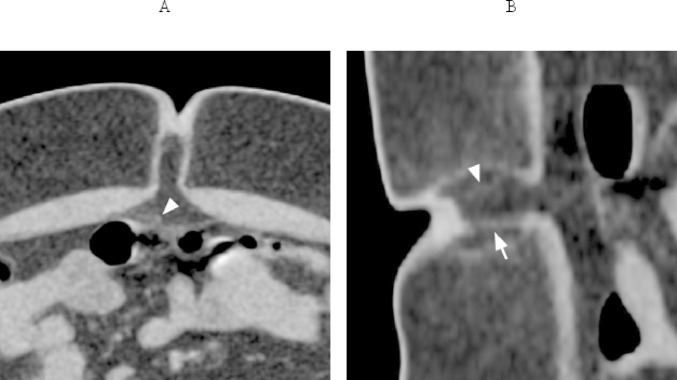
Magnified axial (A) and sagittal (B) CT view of the umbilical canal demonstrates linear soft-tissue structures within the preperitoneal fat tract, corresponding to the ligamentum teres (arrowhead) and the median umbilical ligament (arrow)
